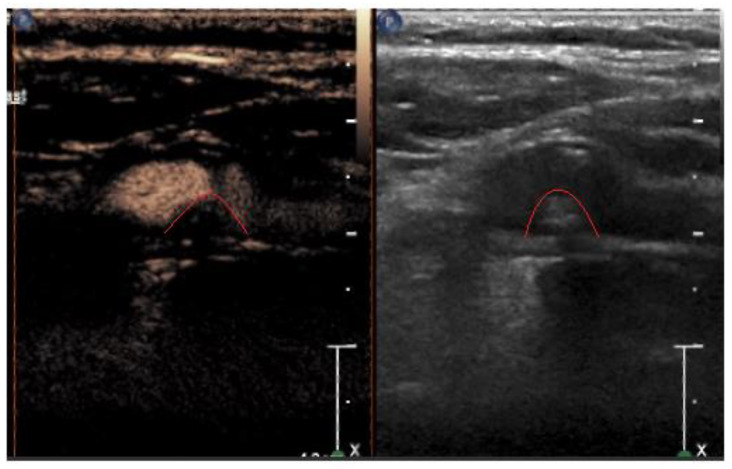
Representative CEUS (left) and grayscale ultrasound (right) images of a grade 1 plaque. The red curved line shows the plaque margins.
